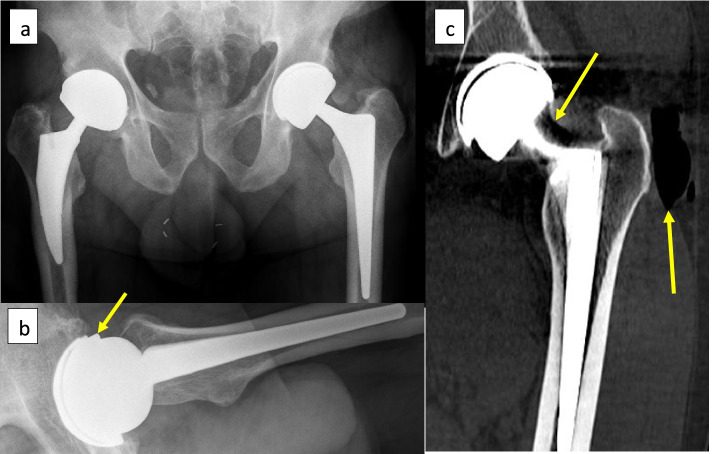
a Anteroposterior pelvic revealing a thin crescent shaped radiolucency between the acetabular shell and the ceramic liner and pneumarthrosis. b Lateral hip radiograph with the arrow pointing at the displaced liner. c CT coronal view of the left hip demonstrating the thin crescent shaped radiolucency between the acetabular shell and the ceramic liner, 2 arrows are pointing at intra-articular gas and gas around the greater trochanter
